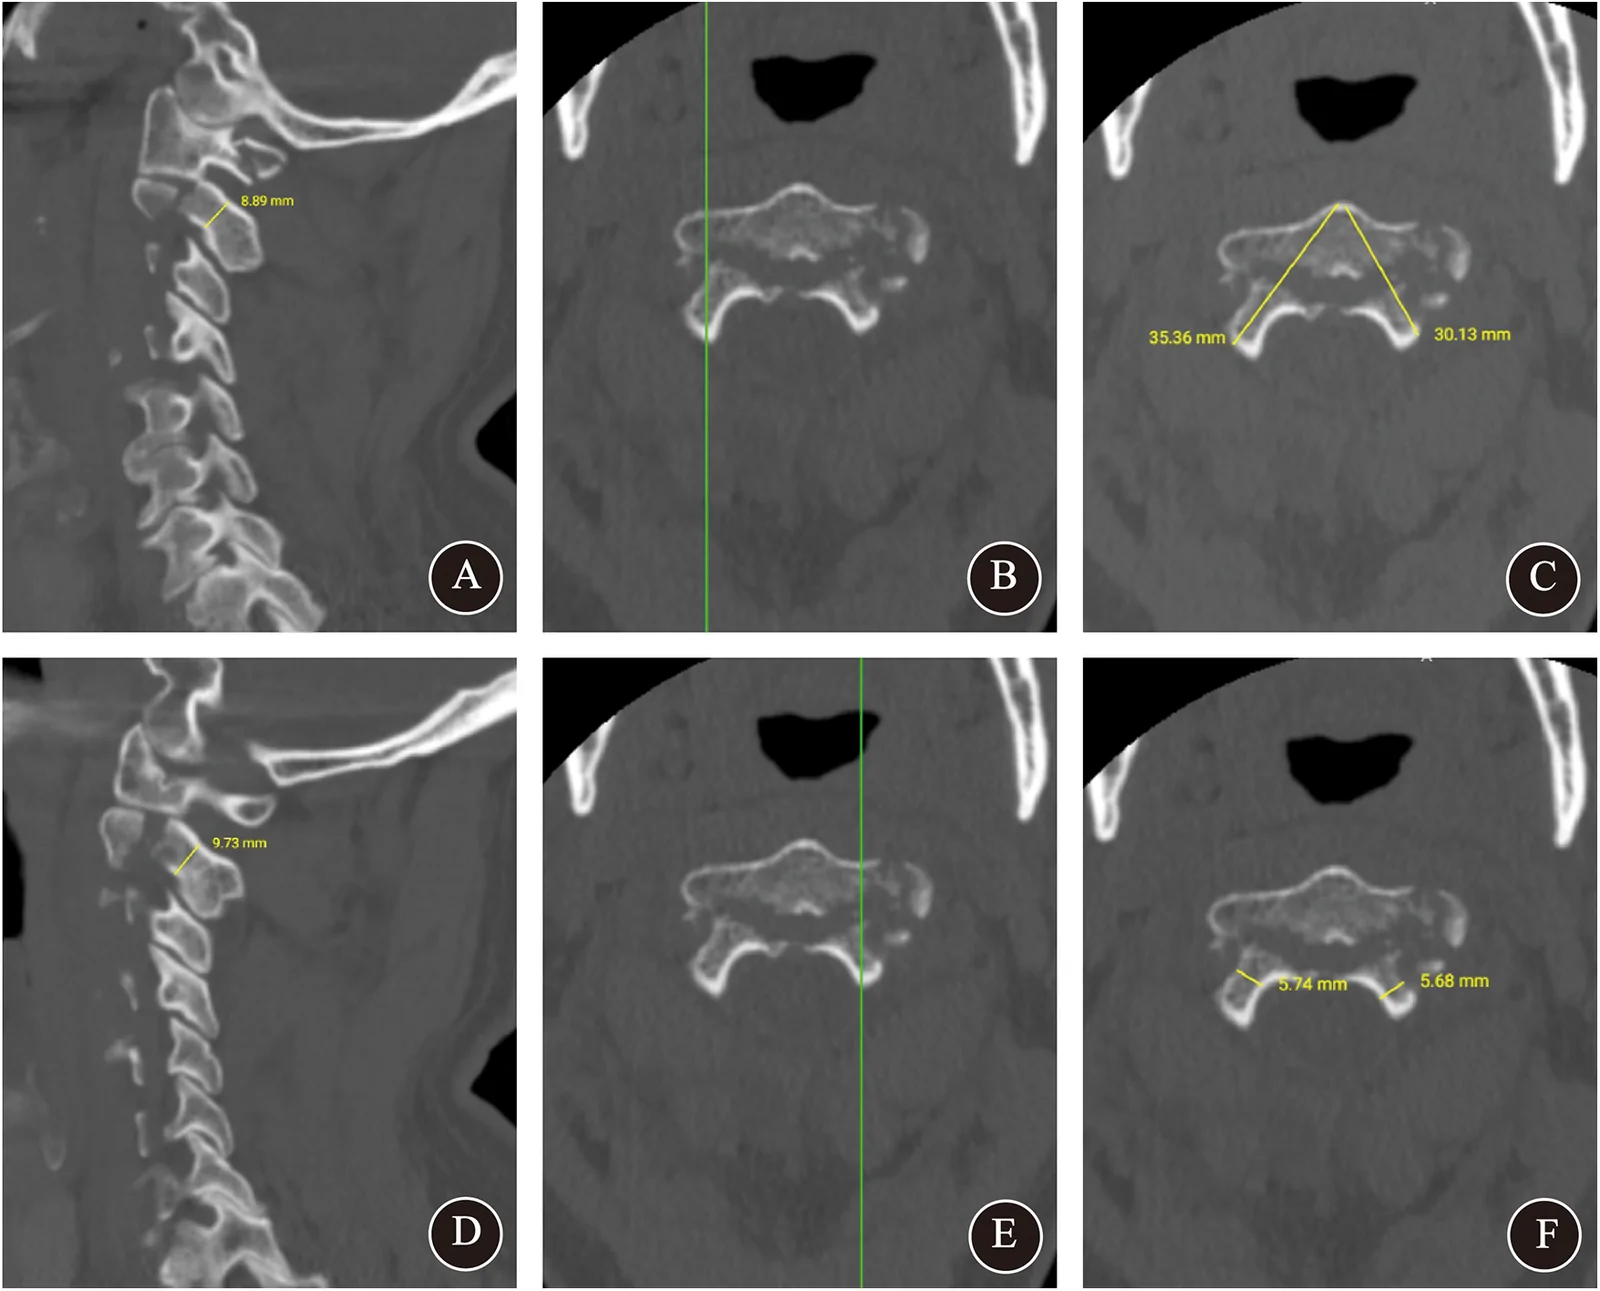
Preoperative cervical CT shows fractures of the right and left pedicles of the axis, with separation and displacement of the posterior wall of the axis. (A,B) The height of the right pedicle measures approximately 8.89 mm. (C) Preoperative planned measurement of pedicle screw length. (D,E) The height of the left pedicle measures approximately 9.73 mm. (F) The width measurements of the bilateral pedicles are 5.68 mm on the left and 5.74 mm on the right.
